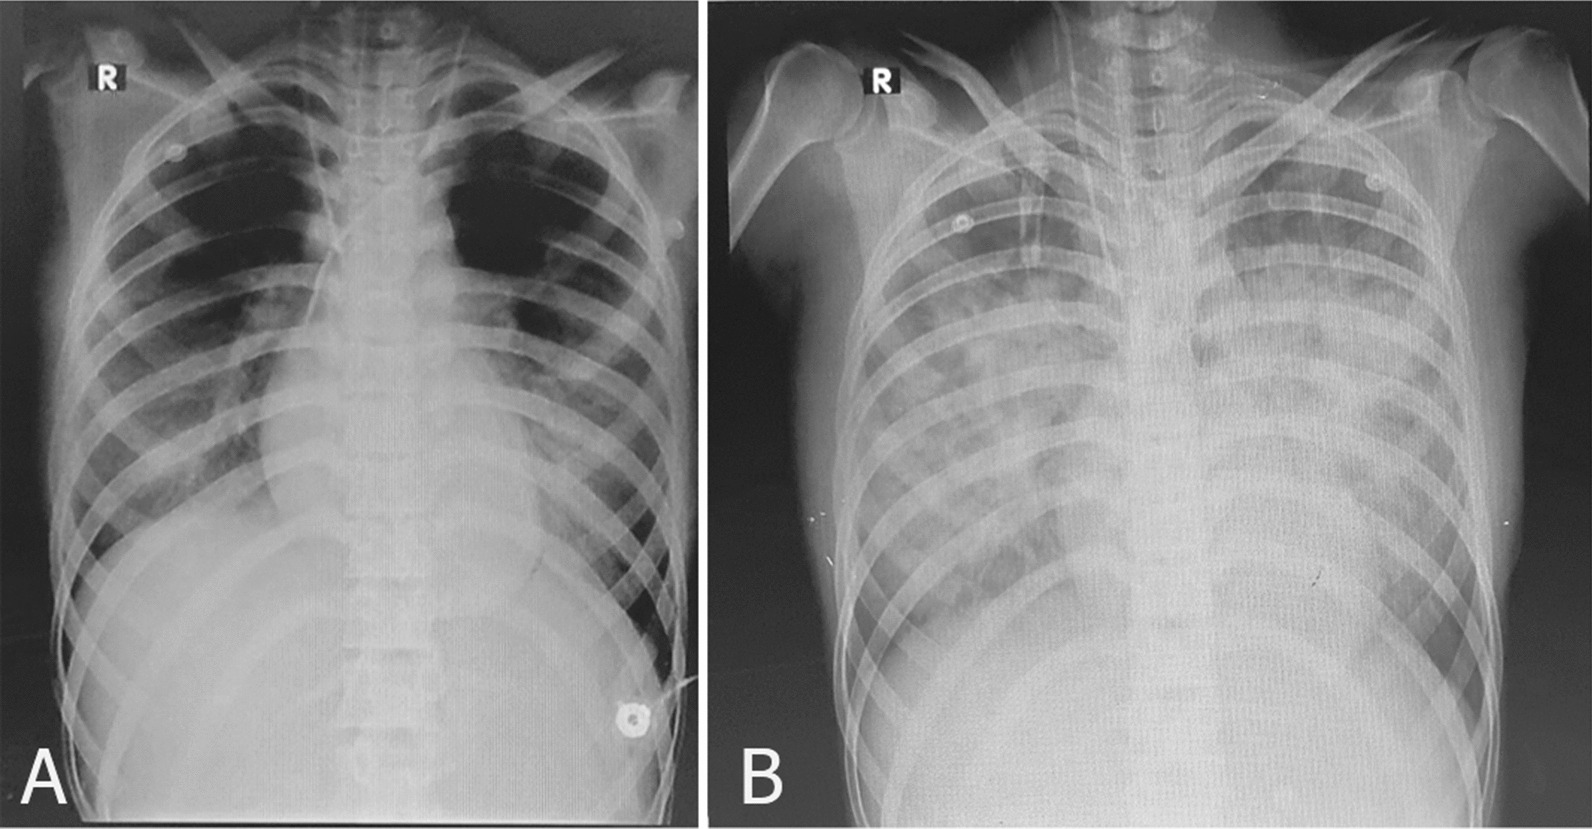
Changes of chest X-ray finding during PICU care, A day 1, B day 3. The progressive changes of chest X-ray and pulmonary edema during PICU care. These changes coincide with changes in the patient’s hemodynamics
